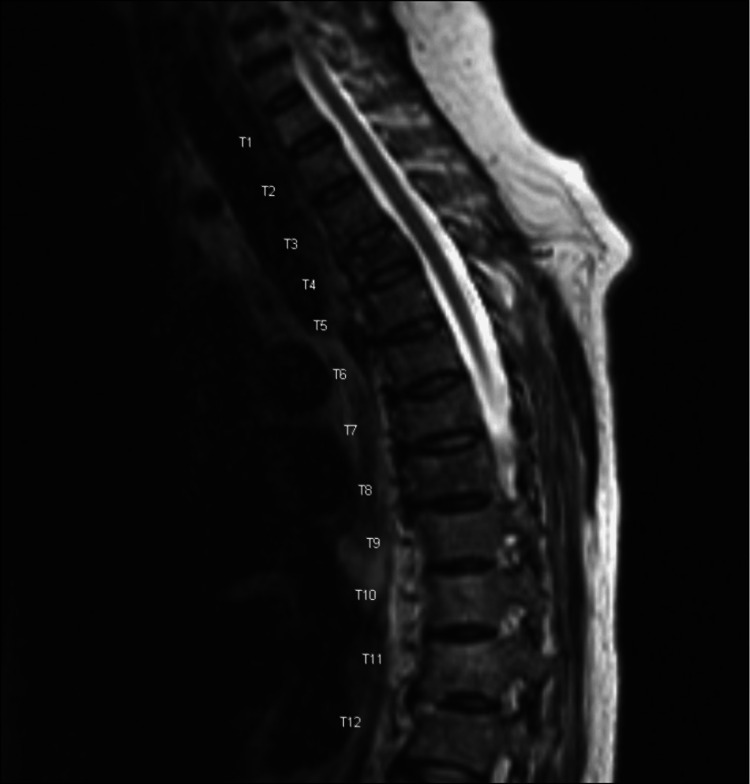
MR thoracic w/o contrast MRI: magnetic resonance imaging Pathologic compression fracture of T4 with 25-50% height loss with mild posterior bulging of the vertebral body without evidence of significant central canal narrowing. Pathologic compression fracture of T7 with less than 25% height loss
